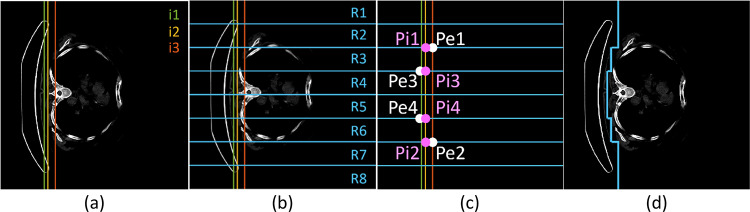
Stretcher removal I: margin location. a Location of the vertical lines that separate the stretcher from the body of the patient: in the central zone (i1, green), in the curve that goes from the central zone to the ends of the stretcher (i2, yellow), and at these ends (i3, red). b Horizontal division of the slice in eight regions of identical size (R1–R8, blue). c Intersection points from Pe1 to Pe4 and Pi1 to Pi4 shown over the correspondent vertical and horizontal lines (CT scan is not included for getting an easier visualization). d Margin (blue) separating the stretcher from the skeletal area shown in the CT slice
